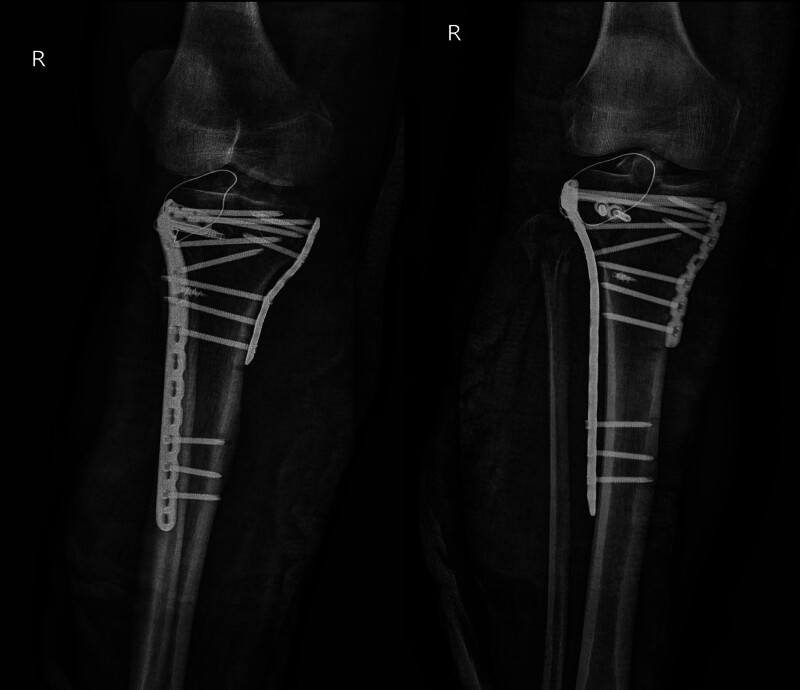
Postoperative radiography showed that the plateau articular surface achieved good reduction, and the metallic anchor and hollow tension screws were rigidly fixed (Fig. 3; date of examination: August 15, 2021). (A) Plain x-ray of the knee with anteroposterior view. (B) Plain x-ray of the knee with lateral view.
